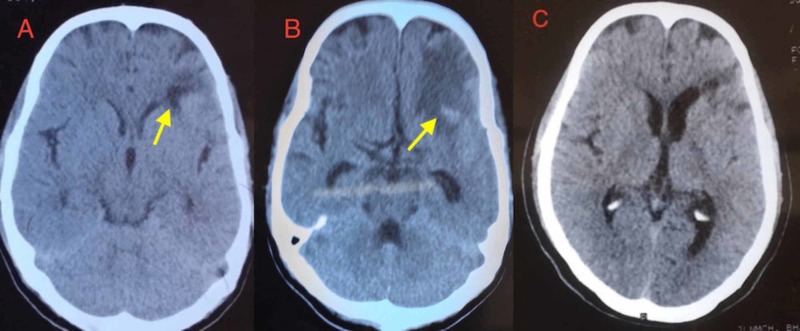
CT scans of the brain Panel A showing hypodense lesion in the left frontal lobe.Panel B showing hypodense lesion with elements of peripheral enhancement in the left frontal lobe. Panel C showing resolution of lesion.
